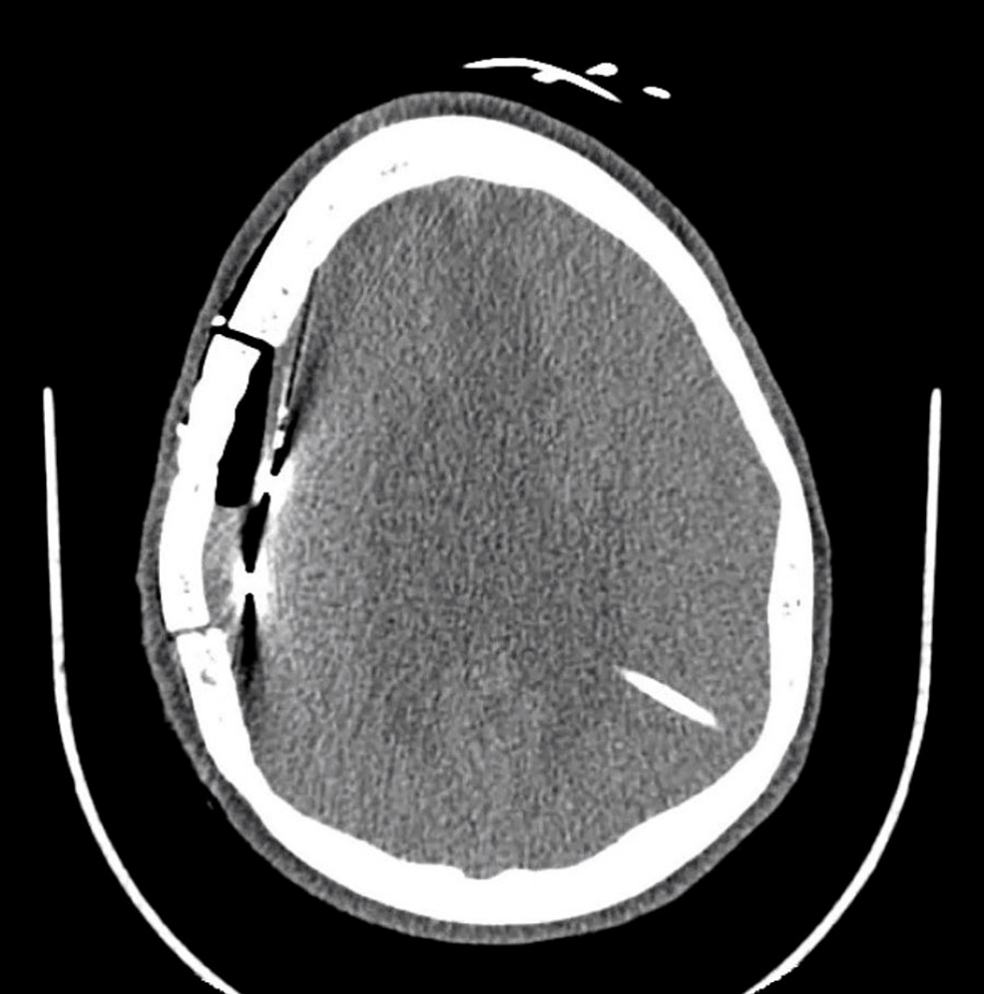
Axial non-contrast CT scan demonstrating a new extra-axial collection, approximately 12 mm in thickness, after placement of 48 contact subdural grids overlying the right frontoparietal area with left ventricular shunt in patient 1 (invasive intracranial monitoring procedure 2 – intracranial grid implantation).
